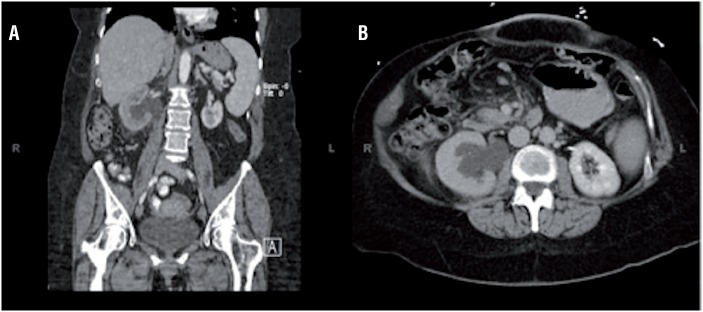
Coronal (a) and axial (b) contrast-enhanced computed tomography revealed moderate-to-severe right hydronephrosis with decreased nephrogram of the right kidney, concerning for high-grade right UPJ obstruction.
